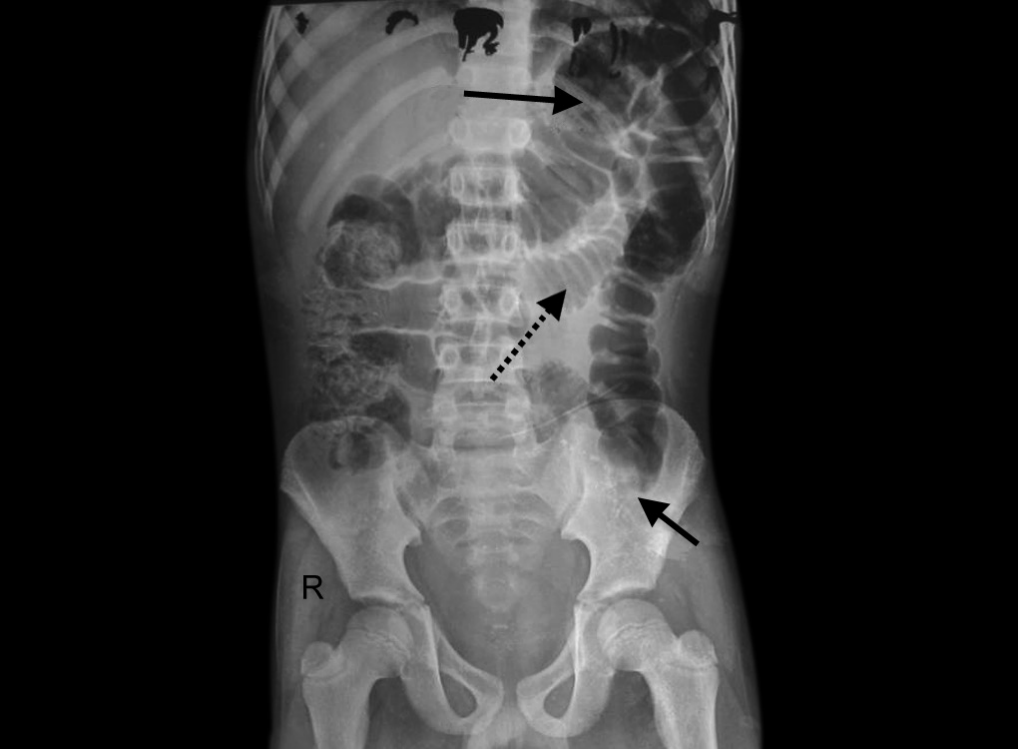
Plain abdomen X-ray taken on postoperative day 1 showing abrupt cut-off at the level of sigmoid colon (small bold arrow) and dilated large intestine (large bold arrow) and jejunum (dotted arrow). There is absence of rectal gas shadow
